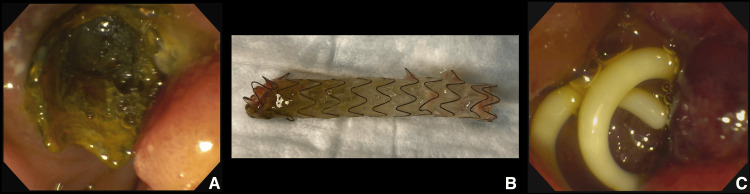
Endoscopic management of the obstructed fully covered self-expandable metal stent (FCSEMS) in the pancreatic duct In the course of the endoscopic retrograde cholangiopancreatography, (A) the FCSEMS (Viabil®; W.L. Gore & Associates, Flagstaff, AZ) was found to be fully obstructed with food, pus, and debris. (B) Following the clearance of all obstructions using a balloon, the FCSEMS was successfully extracted. (C) Subsequently, two short 7 Fr unflanged plastic stents, each with a complete external pigtail (Hobbs Medical, Stafford Springs, CT) were inserted into the main pancreatic duct.
